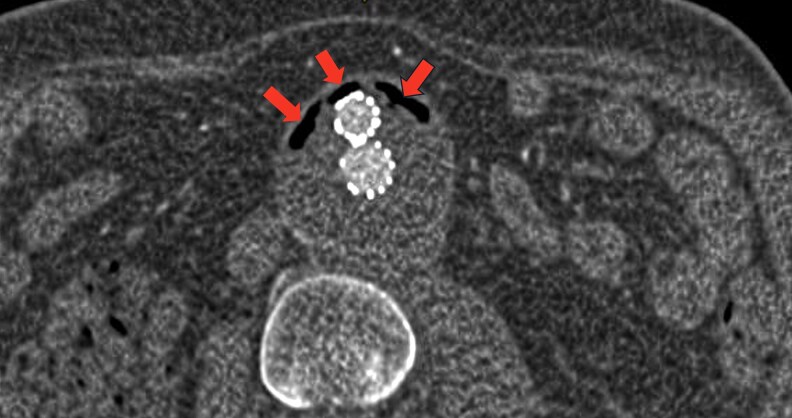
Cross-sectional CTA showing endovascular exclusion of an infrarenal aortic aneurysm with the presence of air within the aneurysm sac indicating an endoprosthesis infection (red arrows).
